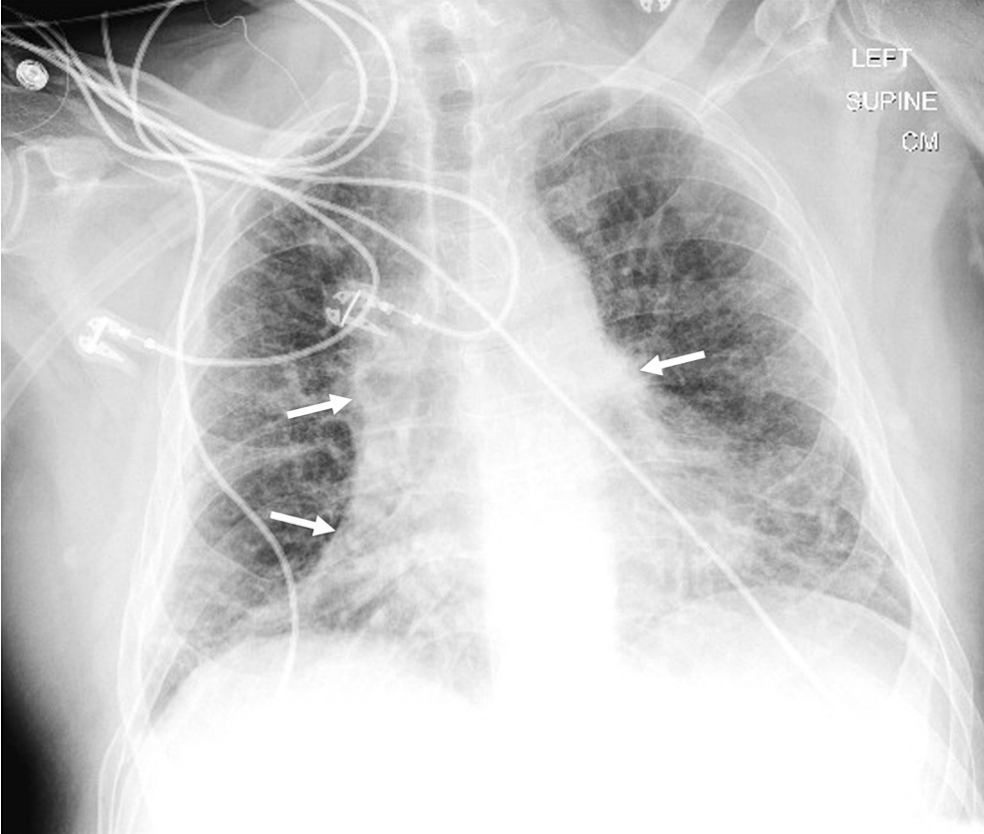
X-ray of the chest Arrows showing bilateral reticulonodular infiltrates and lobar consolidation indicative of pulmonary nocardiosis.
